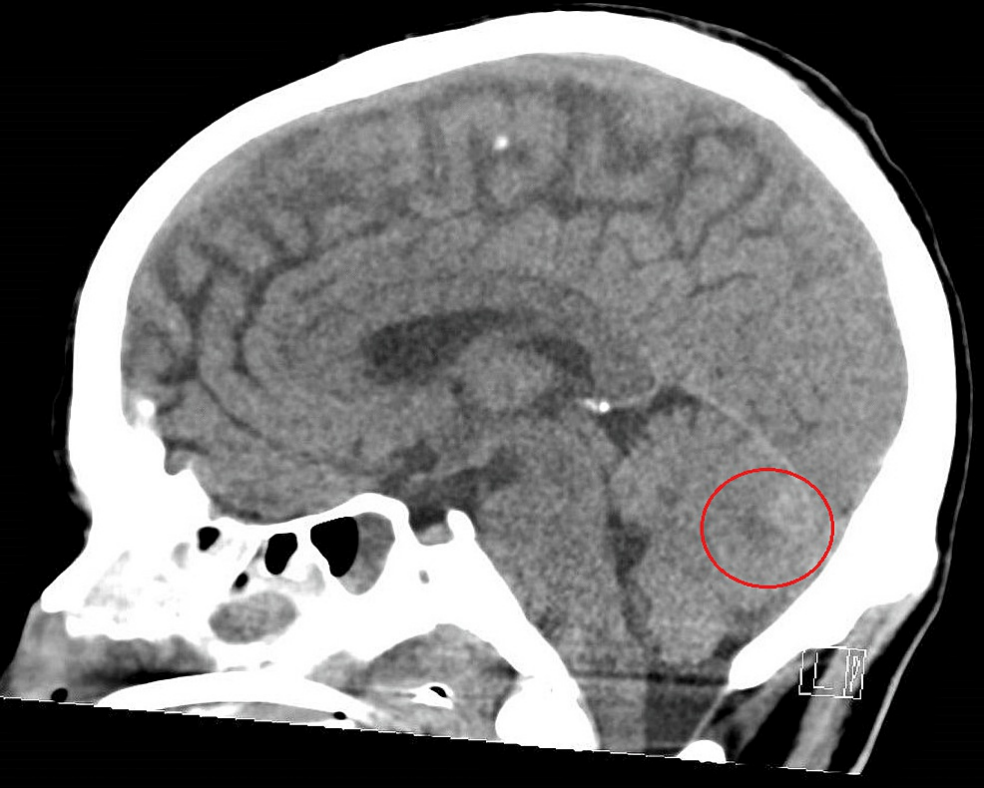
Initial CT Head Initial CT imaging obtained while the patient demonstrated agitation without gross neurologic deficit. Shown is a mid-sagittal slice. Imaging demonstrates a left cerebellar parenchymal hemorrhage (red circle, measured at 3.5 cm x 1.5 cm). Fourth ventricle remains patent. No hydrocephalus.
